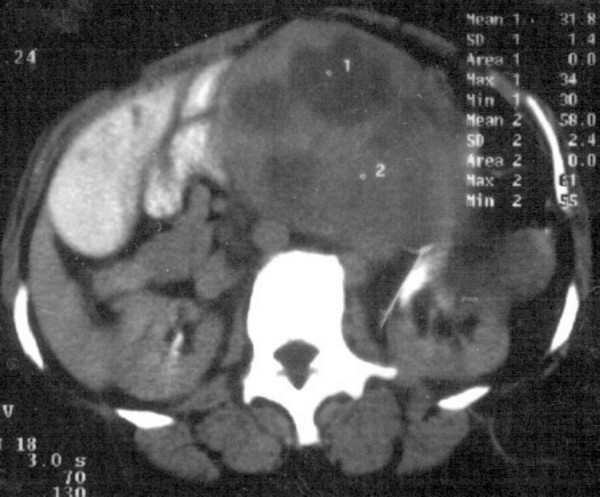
CT abdomen of the patient showing large irregular heterogeneous lesion measuring approximately 16 × 13.6 × 12.1 cm with predominant cystic portions without calcifications.
